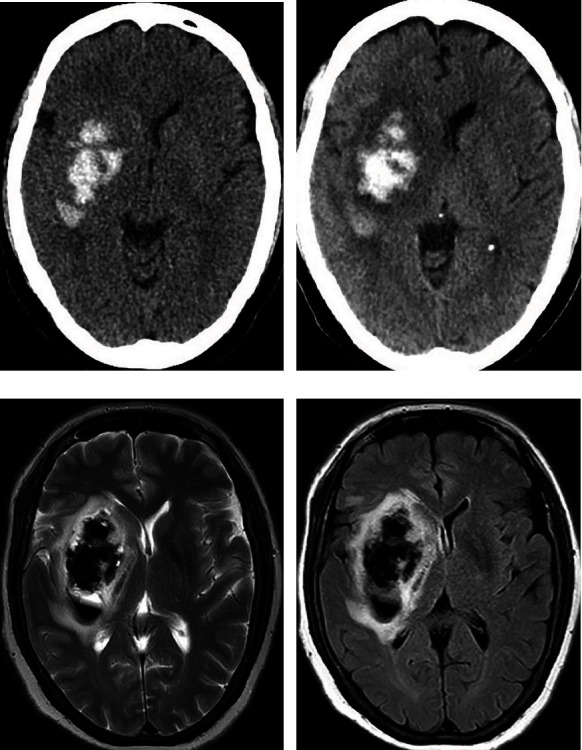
CT scan of a 66-year-old woman who experienced a sudden left limb inactivity accompanied by loss of speech function for 4 hours. There was no intracranial hemorrhage on CT scan before thrombolytic therapy. (a). The non-enhanced brain CT scan obtained immediately after intra-arterial thrombolysis showing a type III hyperdense lesion in the right basal ganglia with a CT value of 55-73 Hu. The right ventricle was significantly compressed. The patient had a marked symptom of neurological deterioration. (b). Follow-up CT scan obtained 24 hours after the end of AR revealed that the contour of the hyperdense lesion located in the right basal ganglia region was slightly expanded, the attenuation was increased, and the surrounding low-density edema occurred, with obvious space-occupying effect. (c). T2WI and D FLAIR obtained 24 hours after procedure showed large patchy low signals in the right basal ganglia region surrounded by high signal. Low signal denotes hematoma and high signal denotes edema.
